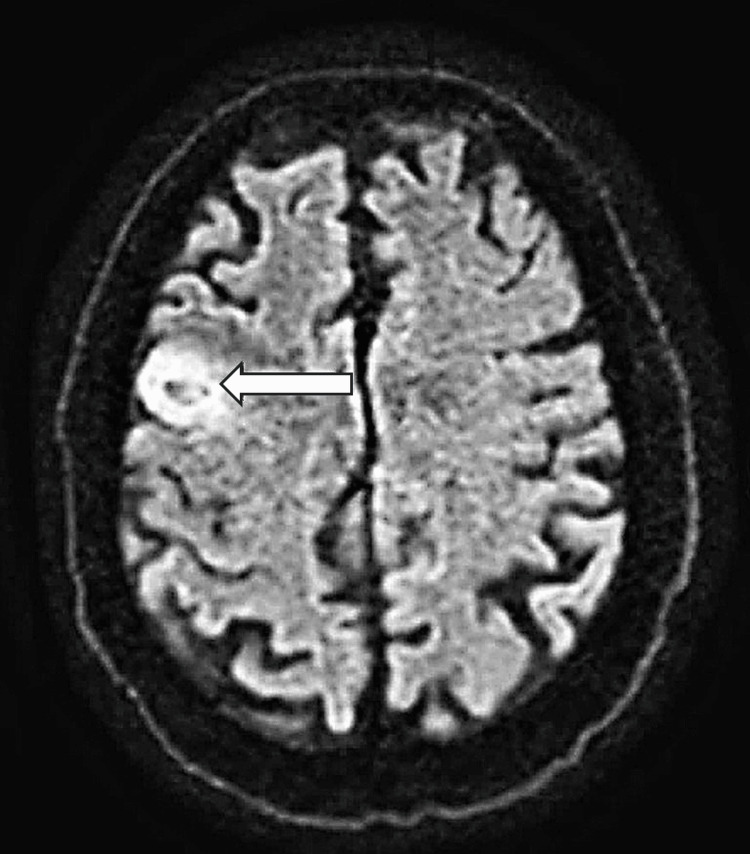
Brain MRI demonstrating a right frontal lobe lesion
